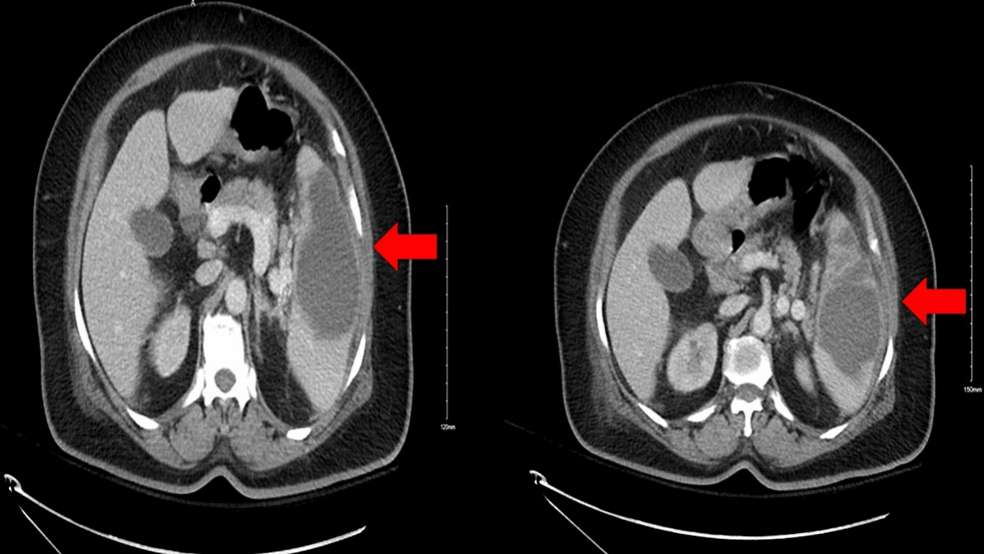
Contrast-enhanced CT of the abdomen upon admission demonstrating a multiloculated collection (red arrows) CT: computed tomography
